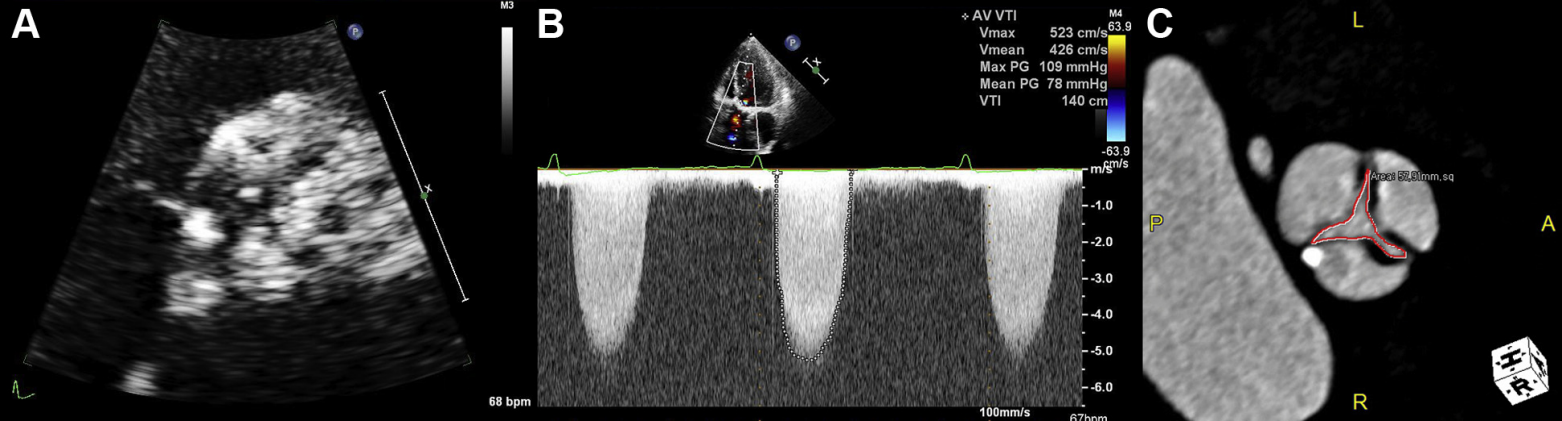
Baseline Transthoracic Echocardiography and Computed Tomography Images Transthoracic echocardiography images demonstrate aortic stenosis with severe degenerative change (A) and an aortic valve (AV) peak velocity of 5.2 m/s and mean pressure gradient of 78 mm Hg (B). Computed tomography image shows AV area of 0.6 cm^2^ by planimetry (C).
